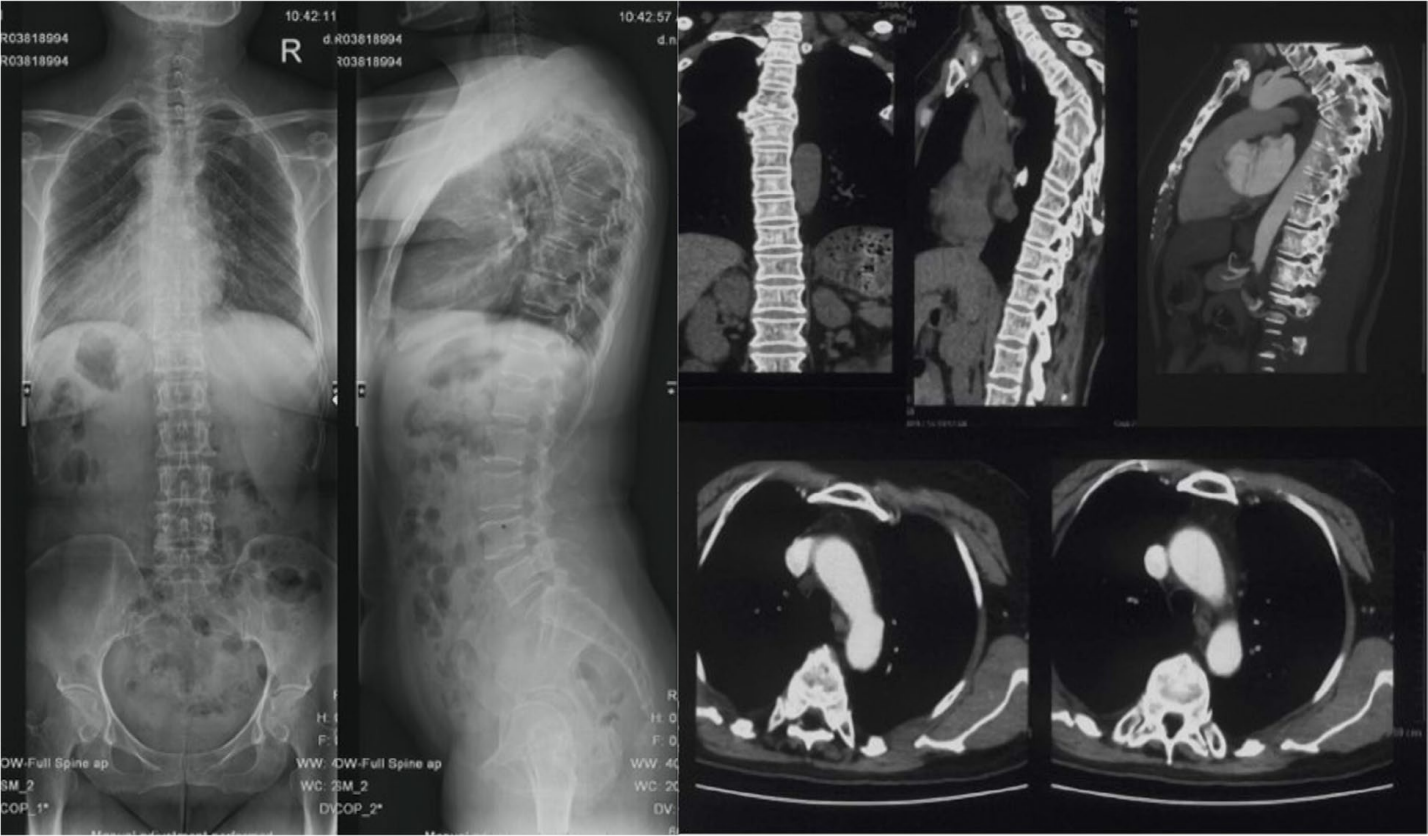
A 64 years old patient presented with weakness and pain of lower limbs. The kyphotic angle of T5-11 was 120° and SVA was measured -1.0 cm. CT scans showed that the aorta was located in vicinity of the rib at apex vertebral level (Grade 4)
